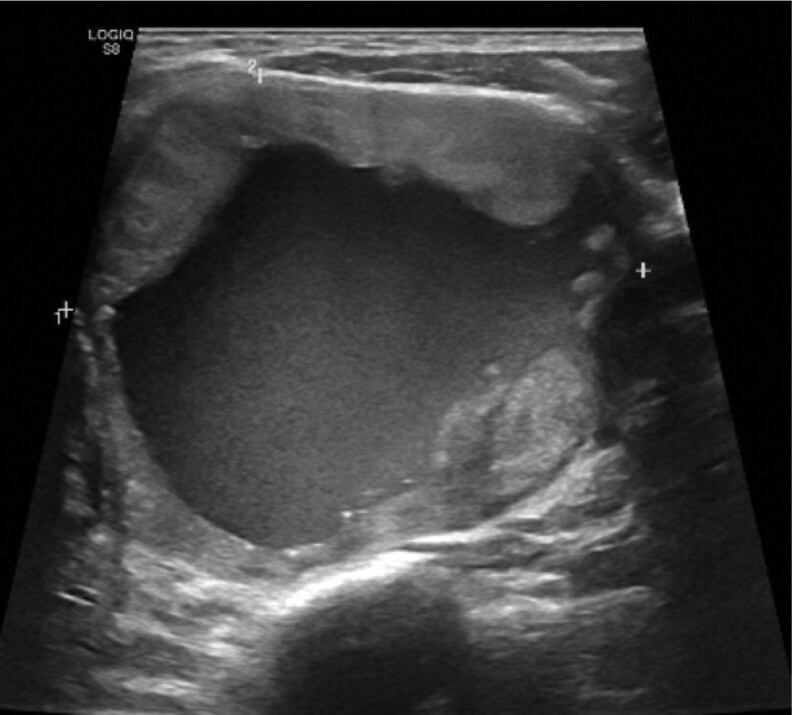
Case #1 preoperative thyroid ultrasound with a 5.9-cm mixed cystic solid left thyroid nodule.
